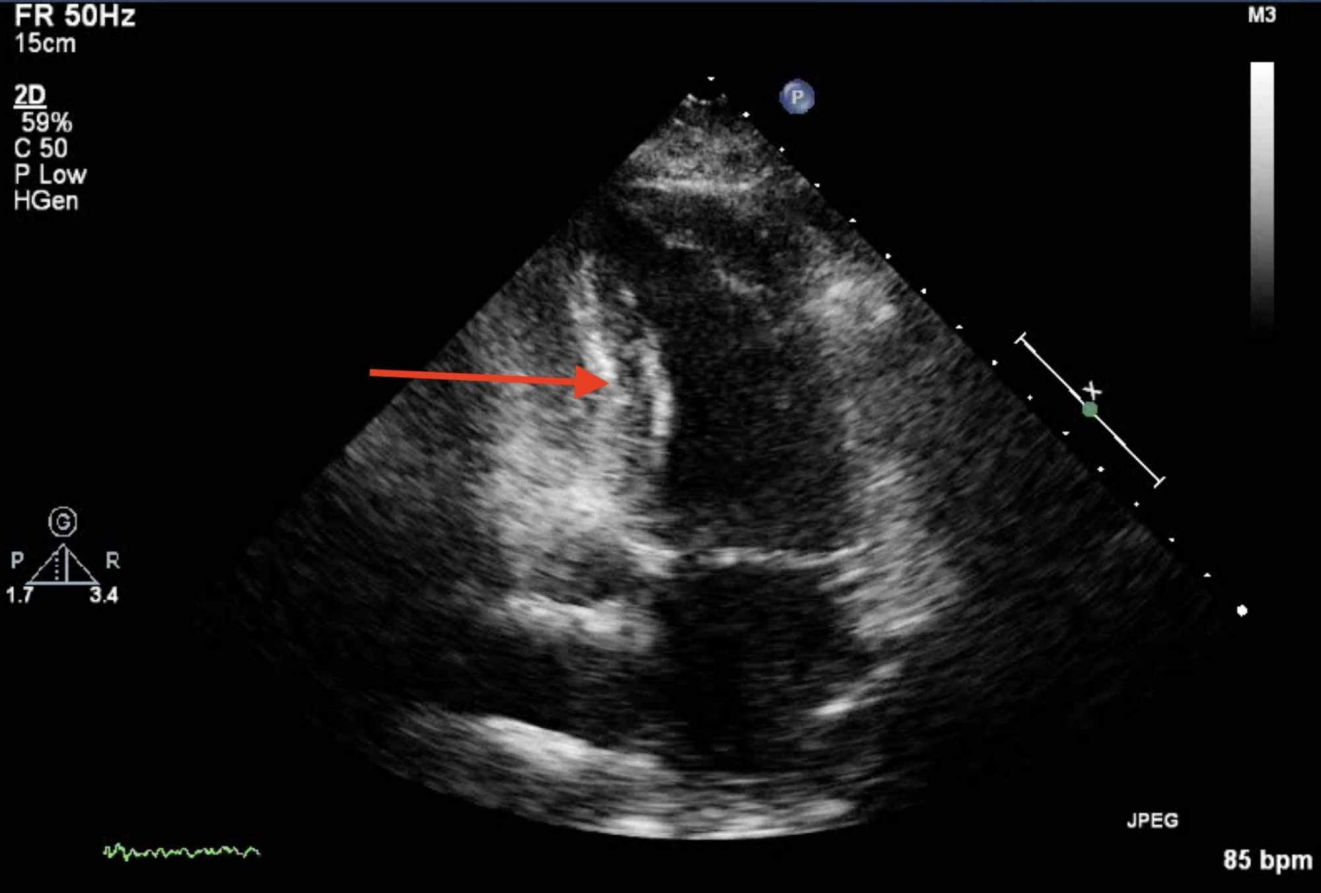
Echocardiogram at follow-up showing improvement of akinesia compared to echocardiogram on admission. Image taken during ventricular contraction. Red arrow demonstrates the area of improved wall motion.
